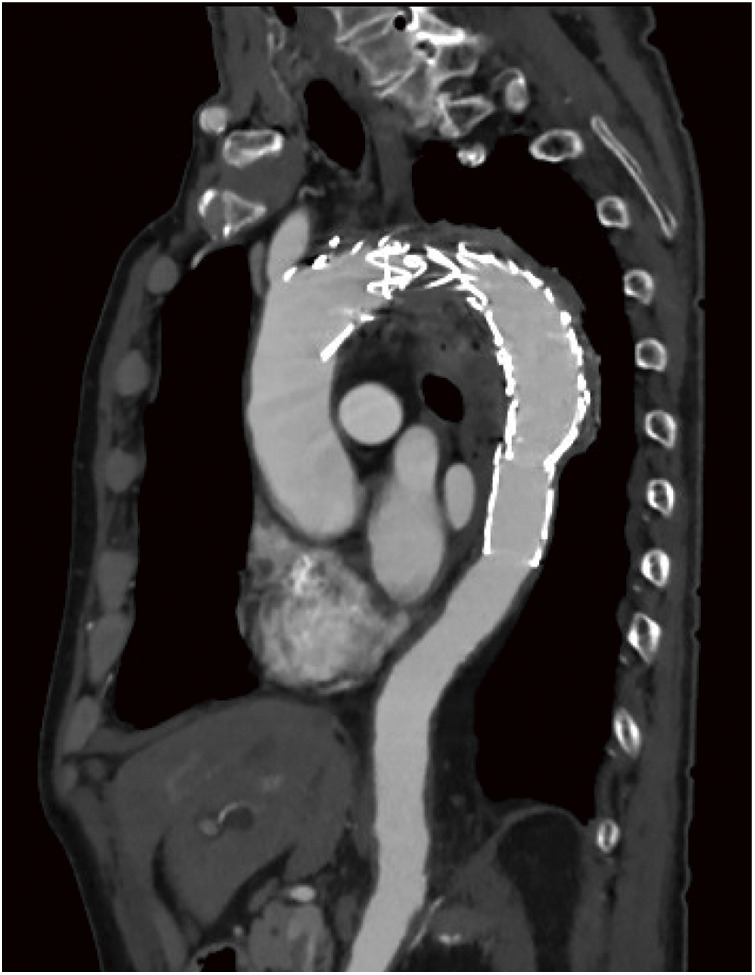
Fig. 5 Final postoperative CT image. Shape of the descending aorta was restored and there was no evidence of rupture. CT: computed tomography
